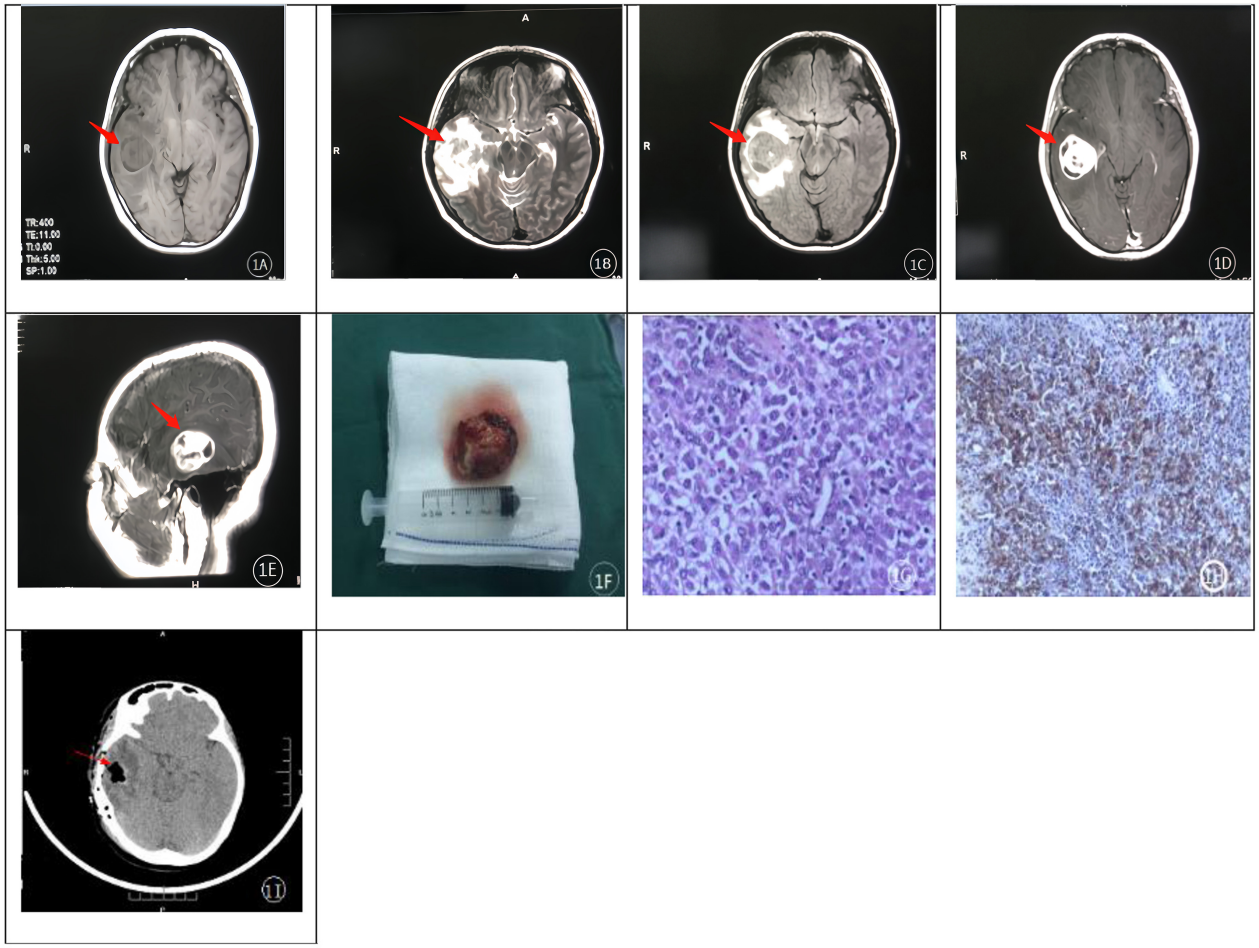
(A–H) shows cranial MRI axial images. T1-weighted (A) and T2-weighted (B) images revealed a mixed signal intensity in the right temporal region, with a slightly isointense signal on FLAIR imaging (C). Extensive perilesional edema was observed on both T1- and T2-weighted sequences. Contrast-enhanced scans (D, E) demonstrated homogeneous enhancement of the lesion, with a small patchy cystic area within it. Pathological sections (G, H) showed eccentric, rhabdoid nuclei, prominent nuclear atypia, frequent mitotic figures, and multifocal necrosis. (F) shows an intraoperative photograph of the tumor resected during the initial surgery. 1 (I) shows the postoperative CT scan following tumor resection.
